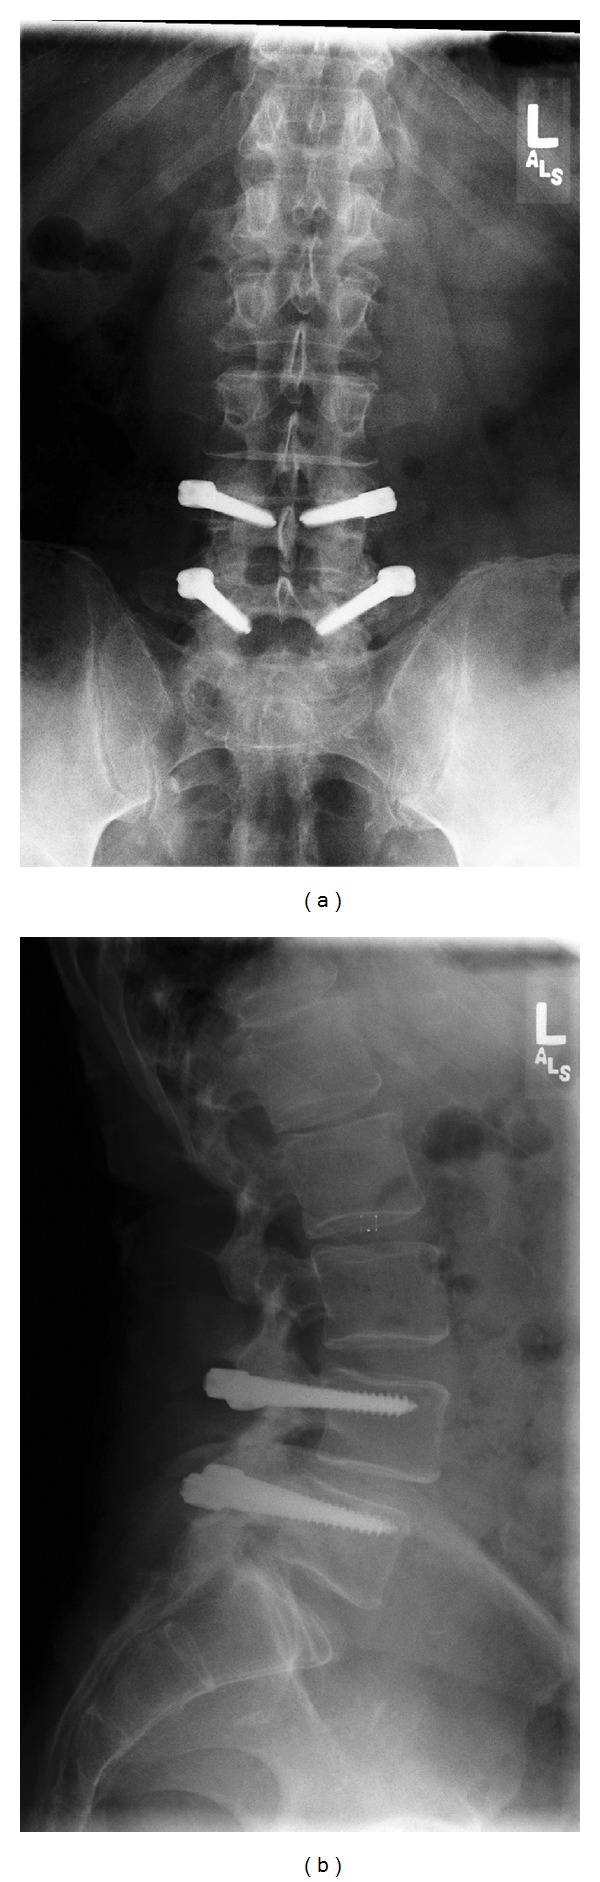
(a) and (b) Postop. anteroposterior and lateral radiographs at 1 year showing instrumentation intact and disc height comparable to preop.
